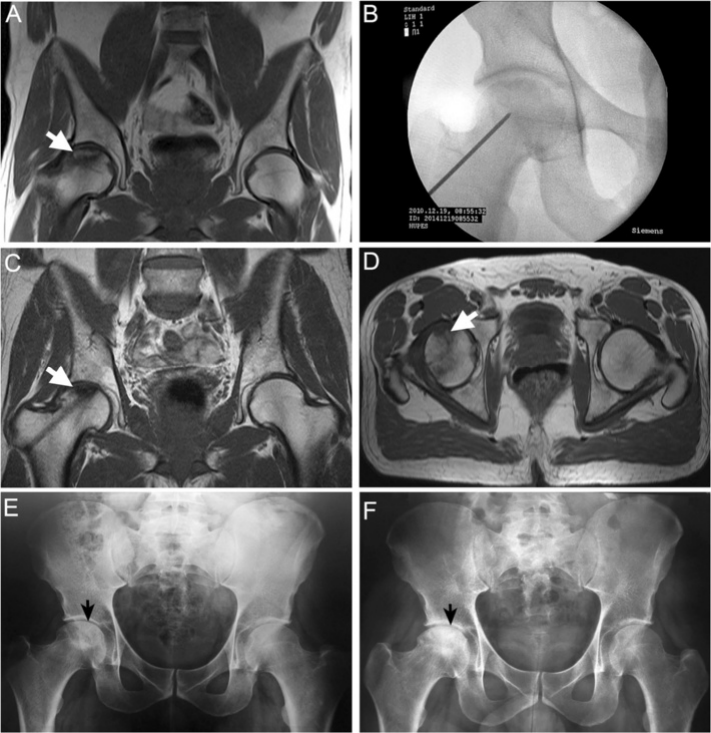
Radiographic sequence from a representative case of a symptomatic 44-year-old man with ONFH successfully treated with BMMC implantation. a Coronal T1-weighted MRI scans of the right hip before surgery show osteonecrotic lesion in Ficat IIB stage. The osteonecrotic lesion is surrounded by sclerotic low-signal margin (*arrow*) with subjacent marrow edema. b Anteroposterior X-ray radiograph showing minimally invasive decompression. The trephine was introduced under fluoroscopy through the greater trochanter into the femoral head. The BMMCs were injected through the trephine into the necrotic zone. c, d Twelve-month postoperative coronal (c) and axial (d) MRI (T1-weighted) scans after autologous BMMC implantation showing the channel, decreased bone edema and changes of signal in the superior part of the femur. Axial MR image (d) shows well-delimited marrow region of increased signal intensity (*arrow*) within the necrotic area and hypointense line around them due to reparative new bone. e, f Preoperative (e) and 60-month postoperative anteroposterior (f) radiographs of the same hip showing the femoral head has maintained the sphericity (*arrow*)
